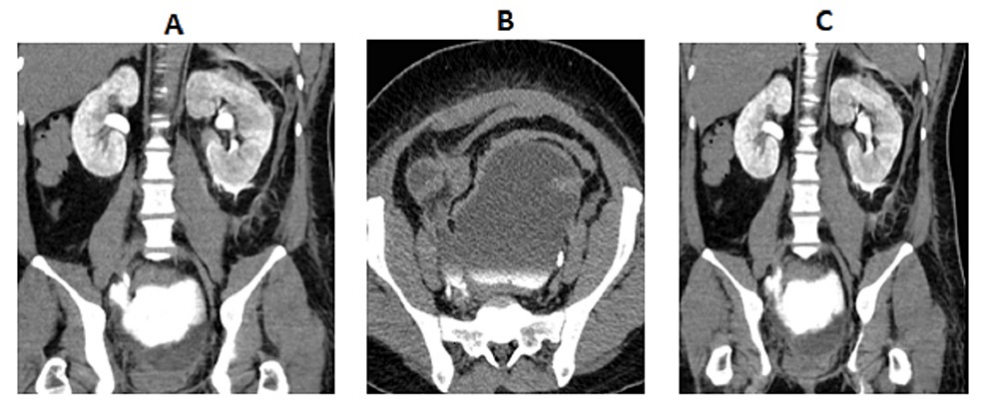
Coronal CT with IV contrast showing right distal ureteric rupture with pooling of contrast from the right side filling up pelvic collection CT, computed tomography
